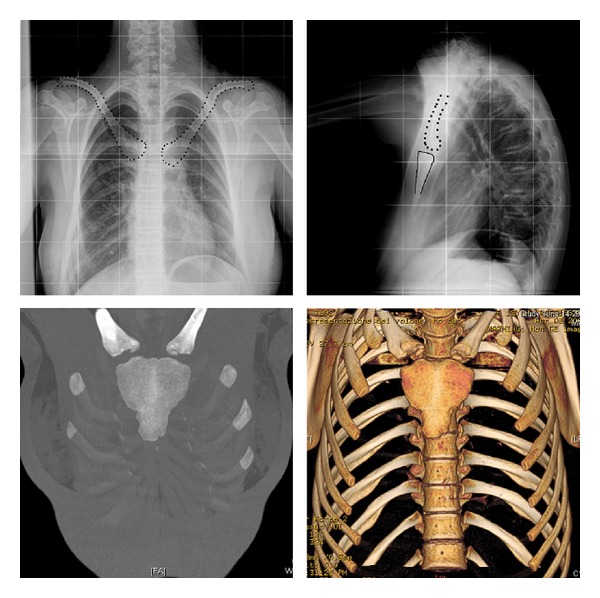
In the X-rays, the clavicles appear with a more vertical course (clavicles dotted line, sternum continuous line). The computed tomography scan with three-dimensional reconstruction documents the abnormal development of the manubrium, the presence of a hypoplastic body, and the absence of the xiphoid process. There is also an alteration of the sternoclavicular joint.
